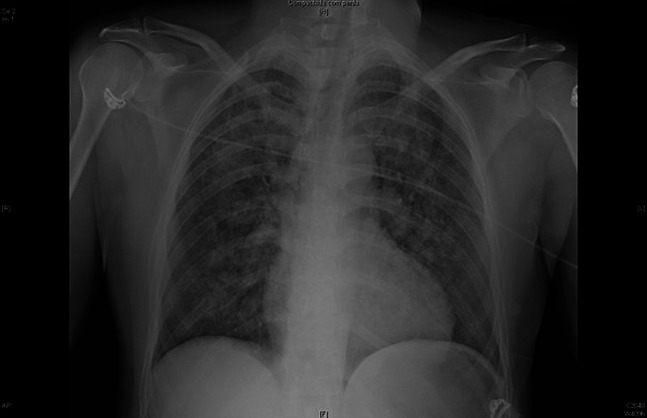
Antero-posterior chest radiography showing a diffuse interstitial and alveolar infiltrate.
